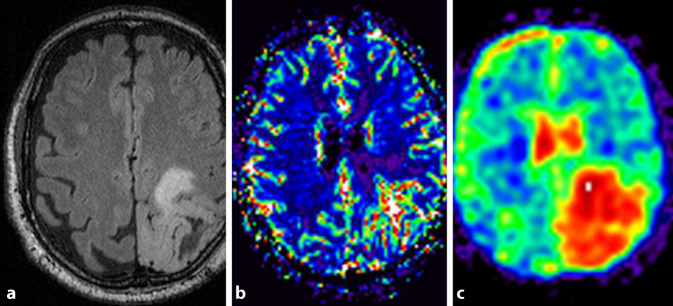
Multiparametric MRI protocol including ^23^NA-MRI. A 59-year-old patient with WHO grade II astrocytoma in the left hemisphere with multimodal MRI including DSC-MRI and ^23^NA-MRI at baseline. a Representative axial FLAIR image. At baseline, rrCBV in the tumor was elevated 1.66-fold compared to healthy contralateral white matter (b). Corresponding axial ^23^NA-MRI slice (c) as color-coded parameter map. Relative sodium content within the tumor was 1.99 when compared to contralateral healthy white matter at baseline. At the 22-month follow-up, the time point of progressive disease as determined by RANO criteria, relative sodium content decreased to 1.77 (not shown)
